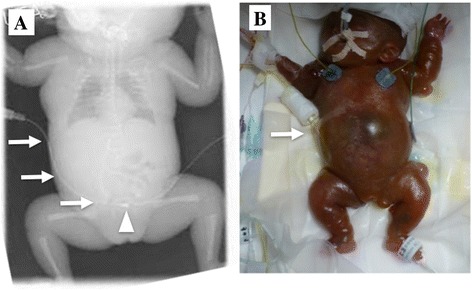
a Abdominal X-ray of the patient on DOL 17. Arrows indicate the percutaneous drainage tube. The tip of the catheter was placed in the Douglas pouch (arrow head). b Photograph of the patient on DOL 21. PD was started by initially inserting a drainage tube. The arrow indicates the initially inserted drainage tube for peritoneal drainage
